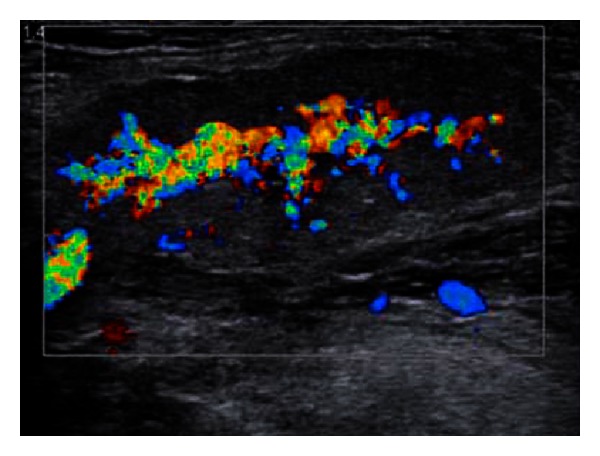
Colour coded Doppler ultrasonography (same case as Figure 1). In the central part of the gland there are vessels (blue and red according to the direction of the blood flow in relation to the transducer). The amplitude and extension of the colour signal are suggestive of hyperaemia (in this case it was an acute inflammation of the submandibular salivary gland).
